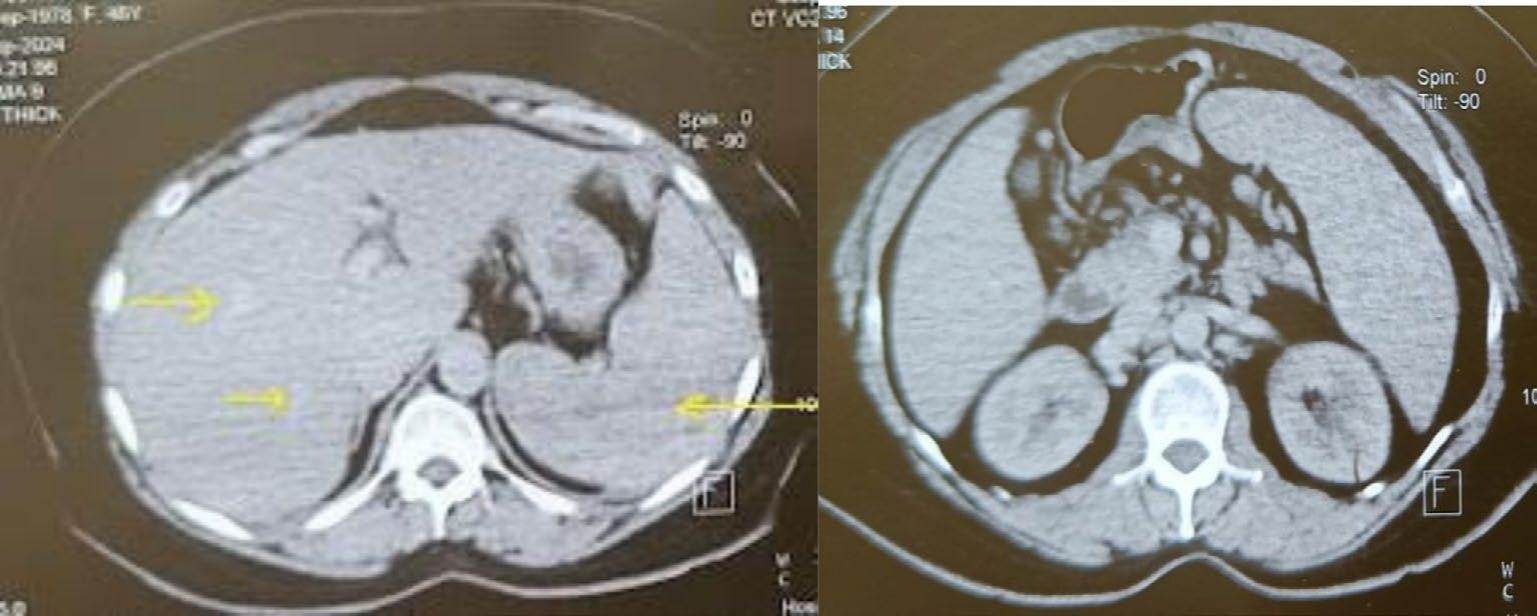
Transverse section on CT showing nodular macro deposits over liver and spleen with abdominal lymphadenopathy (yellow arrows—nodules better appreciated on PET‐CT).
